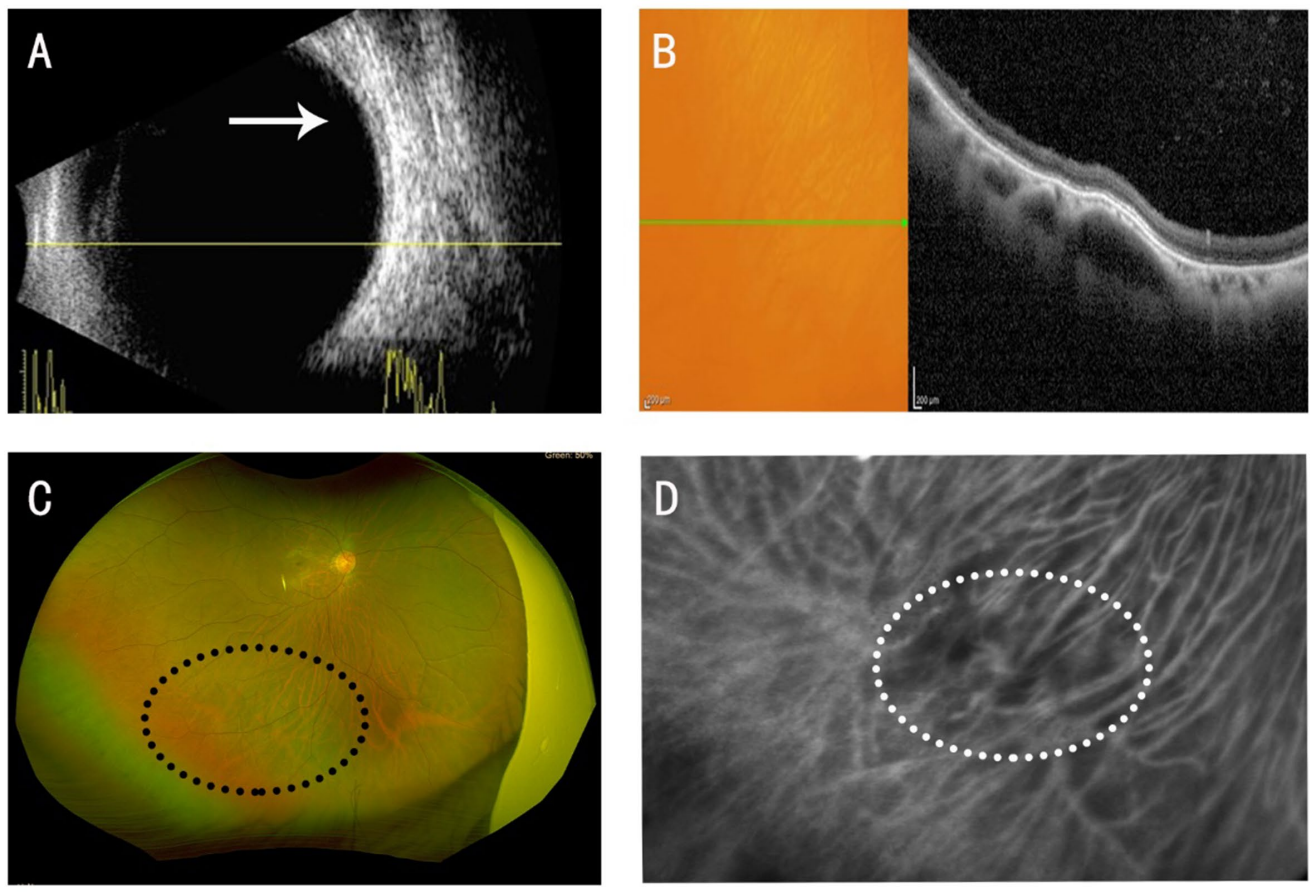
After applying appropriate pressure using an ophthalmic B-scan ultrasound probe to the patient’s eyeball. (A) B-scan ultrasonography: Resolution of the choroidal space-occupying lesion, as indicated by the white arrow. (B) OCT: Significant reduction in the retinal low-reflective cavity. (C) Fundus photography: Disappearance of the brownish-red mass in the inferotemporal region, as indicated by the black circle. (D) Near-infrared imaging: Reduced dilation of the vortex vein ampulla, as indicated by the white circle.
